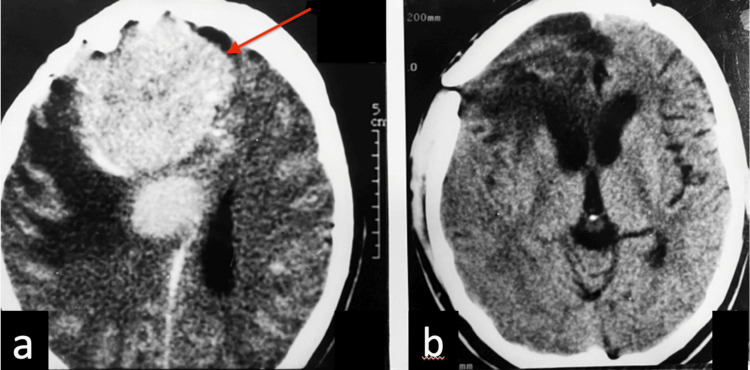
Brain CT findings a: brain CT scan showed tumor recurrence (red arrow) in the right frontal region and a new right parietal lesion; b: postoperative brain CT scan showed no tumor.
